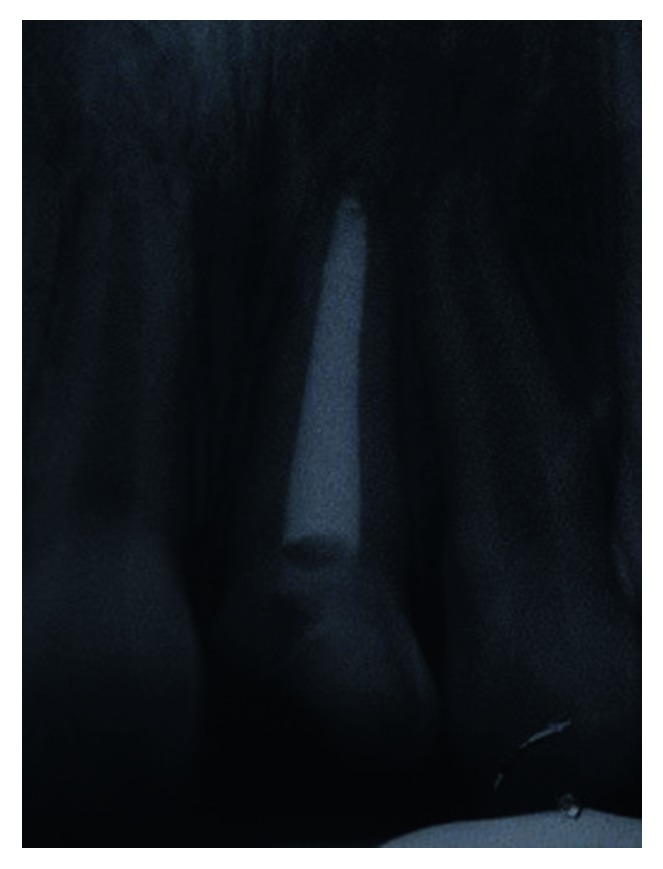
Periapical radiograph of tooth 21 following extrusion prior to reattachment of the fractured fragment of the tooth.
